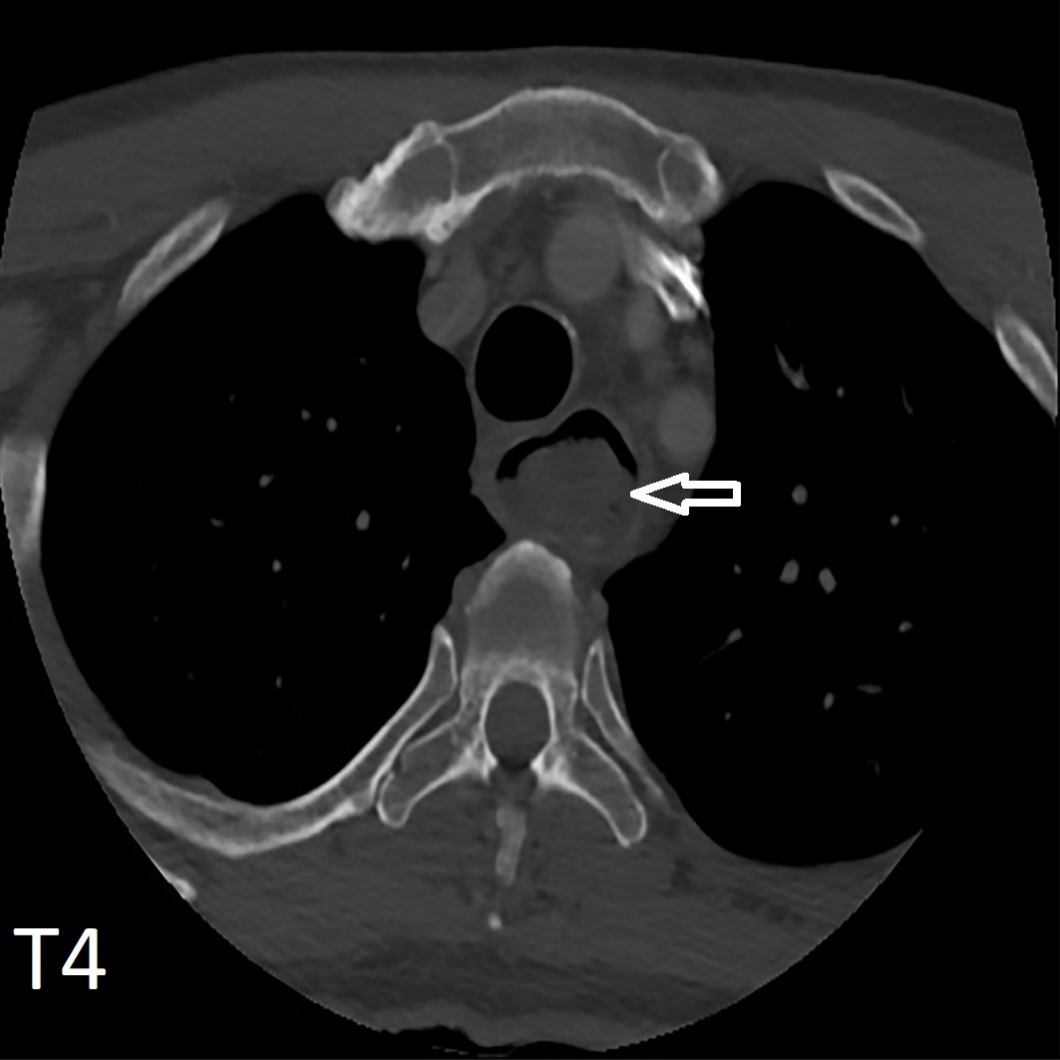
Computed tomography scan (chest) with contrast at T4 level showed long segment dilation of the upper and mid thoracic esophagus with intraluminal heterogeneous material with generalized circumferential thickening of the distal esophagus. T4 (4^th ^thoracic vertebra)
